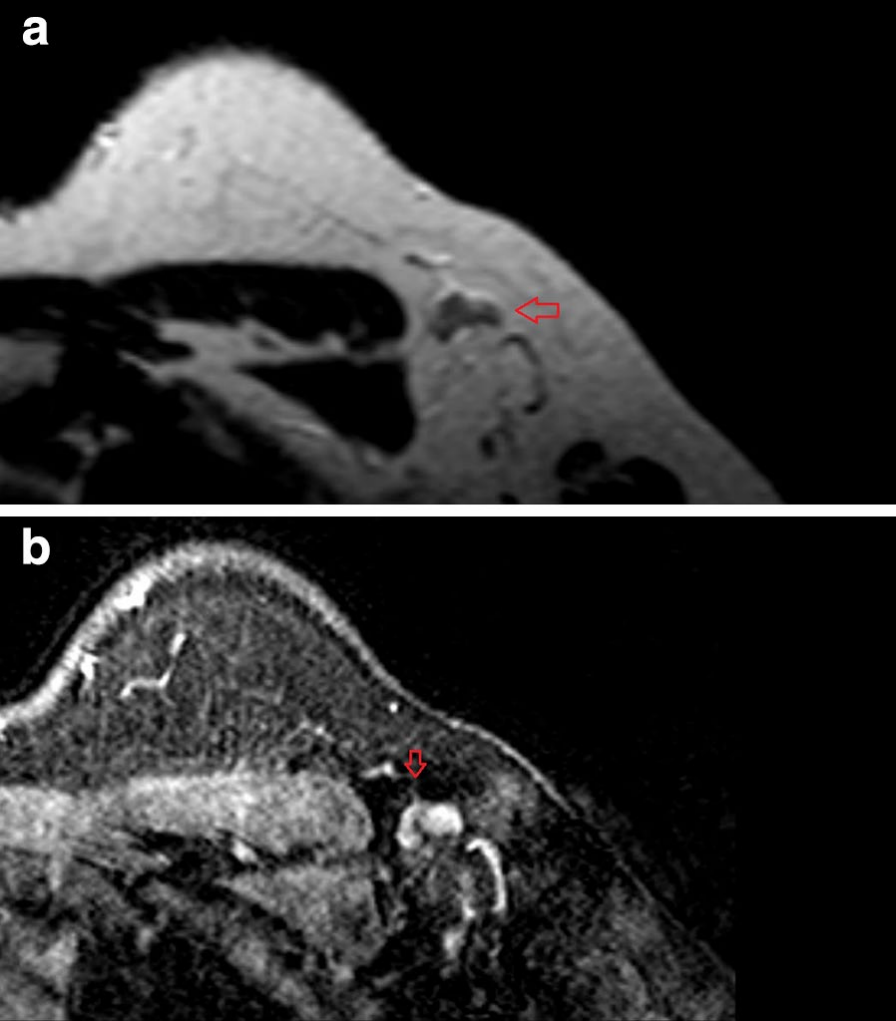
a Axial T2WS-TSE MRI showing an enlarged lymph node on the right axilla. Fatty hilum is absent with an increased asymmetrical cortical thickness. b The lymph node has a small tail on axial post contrast T1WS MRI, this is called “comet tail sign”
